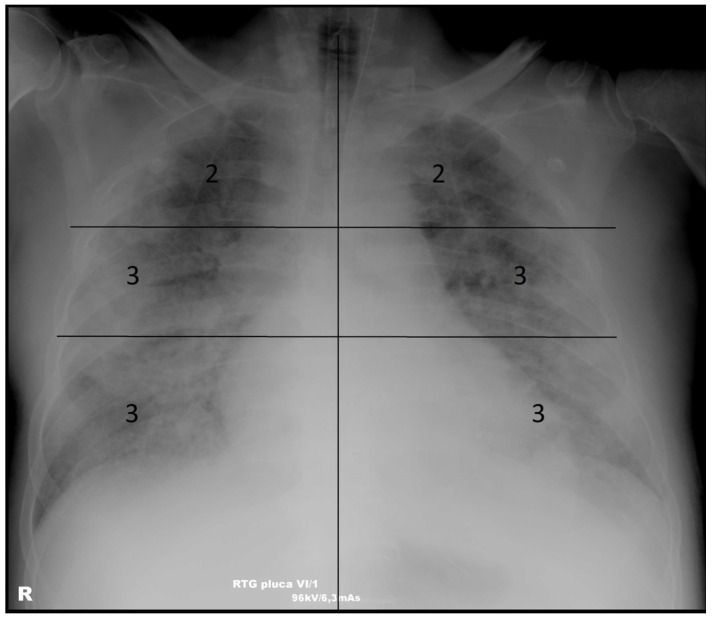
Supine chest X-ray image of a male patient with COVID-19 pneumonia and extensive bilateral airspace disease—modified Brixia score value 16. 2—presence of consolidation involving up to 50% of the lung parenchyma in the assessed zone. 3—presence of consolidation involving 50% or more of the lung parenchyma in the assessed zone.
